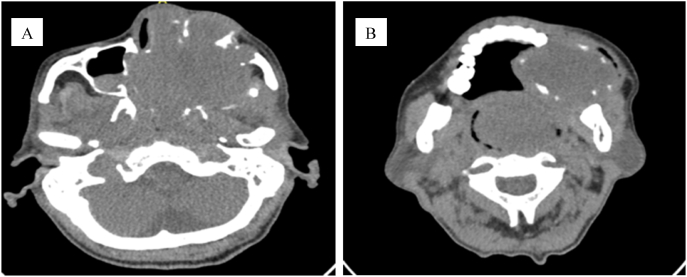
Computed tomography (CT) scan of the paranasal sinus and neck. A, Heterogenous opacification of the left maxillary sinus, bilateral nasal cavities, bilateral ethmoid, sphenoid, and frontal sinuses, extending to the nasopharynx. B, Soft tissue density extended inferiorly to the alveolar ridge of the left maxilla.
